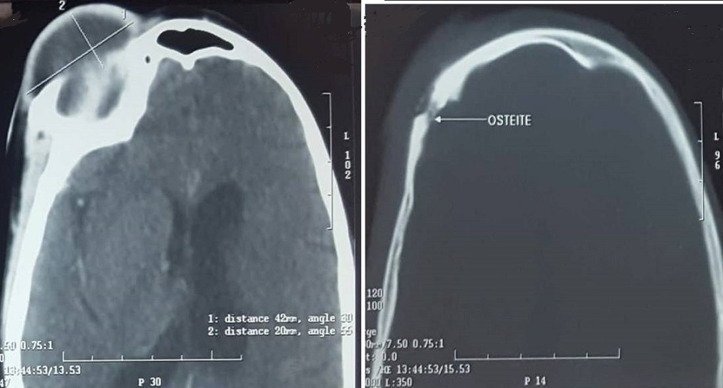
axial brain CT scan showing supra orbital collection and the frontal bone lytic lesion
